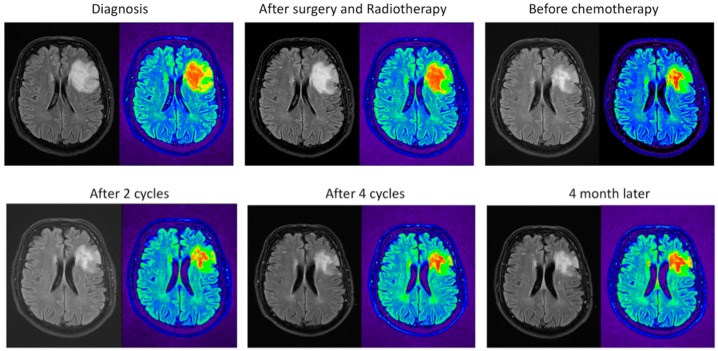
Pseudo-colored shades of gray of the MRI plots. Pseudo coloring was performed by the OsiriX Lite program by using the NIH palette. The slice that is depicted is from the highest tumor density. The highest intensity is colored red and are probably cancer stem cells, while the differentiated cells are colored green.
